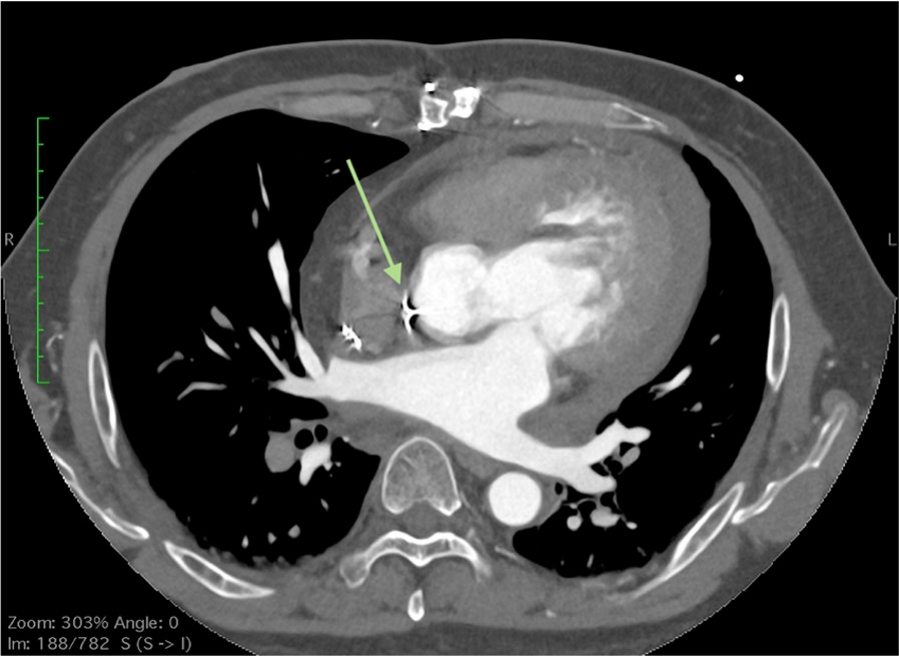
Preoperative computed tomography image to evaluate for cardiogenic shock. A 20-mm pericardial effusion was observed posterior to the left ventricular wall. The atrial lead tip was located proximal to the ascending aorta (green arrow)
